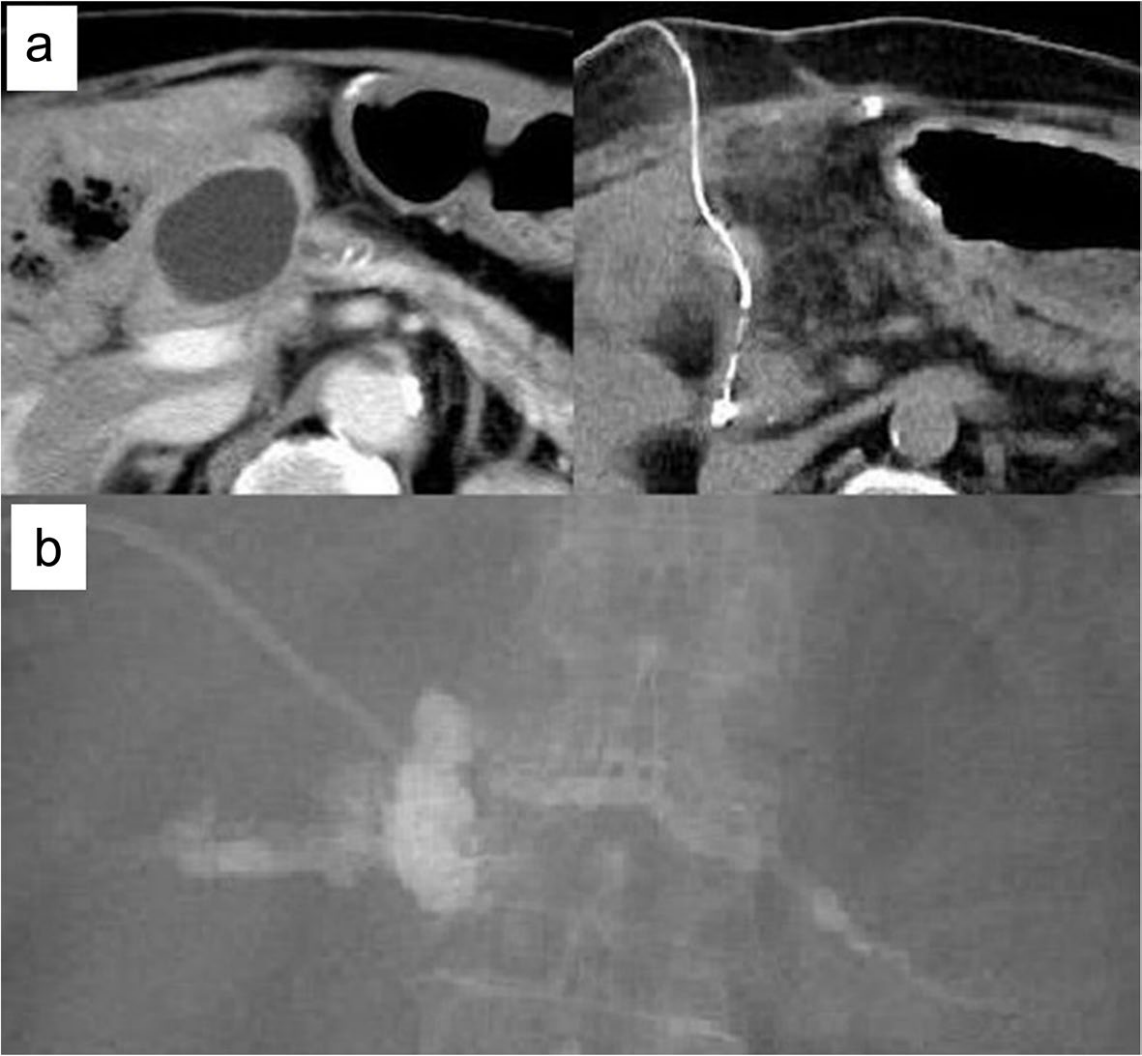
Preoperative computed tomography (CT) and percutaneous fistulography findings. a CT indicates failure of the pancreaticojejunal anastomosis with pseudocyst formation, including pooled pancreatic juice and dilation of the main pancreatic duct (MPD) of the remnant pancreas. b The percutaneous fistulography shows MPD that does not communicate with the Roux-limb
